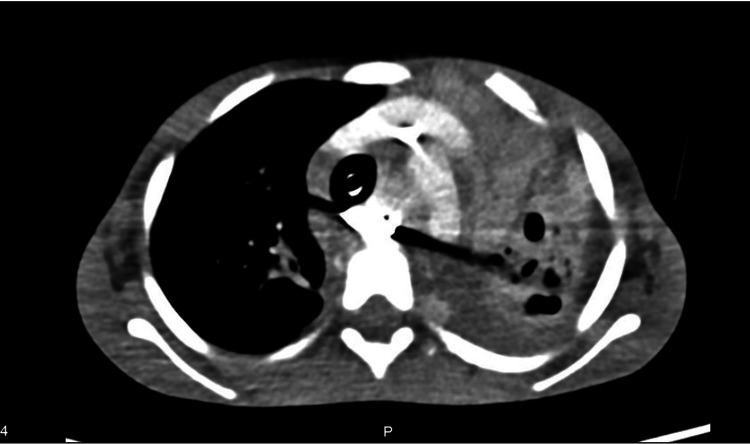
Axial CT scan of the chest after intubation CT scan with a foreign body impacted in the medium third of the esophagus, signs of chronic mediastinitis, chronic left lung atelectasis, and bronchiectasis.
